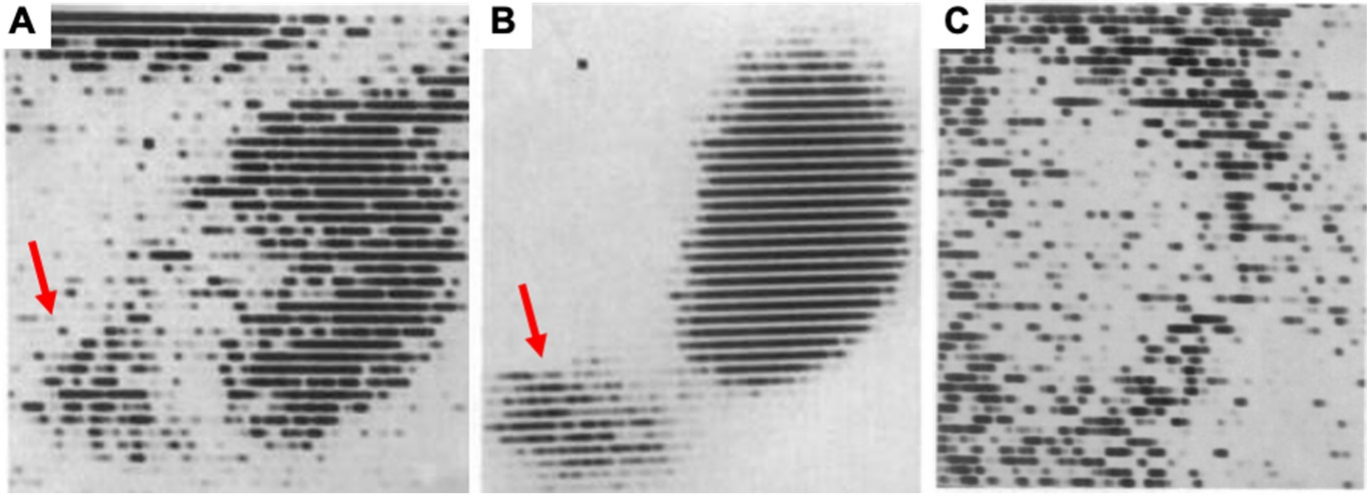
First renal scintigraphy with Chlormerodrin (^203^Hg), showing (A) rather less clear images shortly after transplantation, while in a long-term follow-up after 63 days (B), a “great improvement in the renal contours“ was noted by Figueroa et al 1. Red arrows indicate uptake in the bladder. In (C), a renal scan of patient 5 days after transplantation is displayed and the scan showed “negative contrast” with almost no uptake in the kidney. The patient died 8 days later. Adapted with permission from 1, copyright 1965 NEJM Massachusetts Medical Society.
